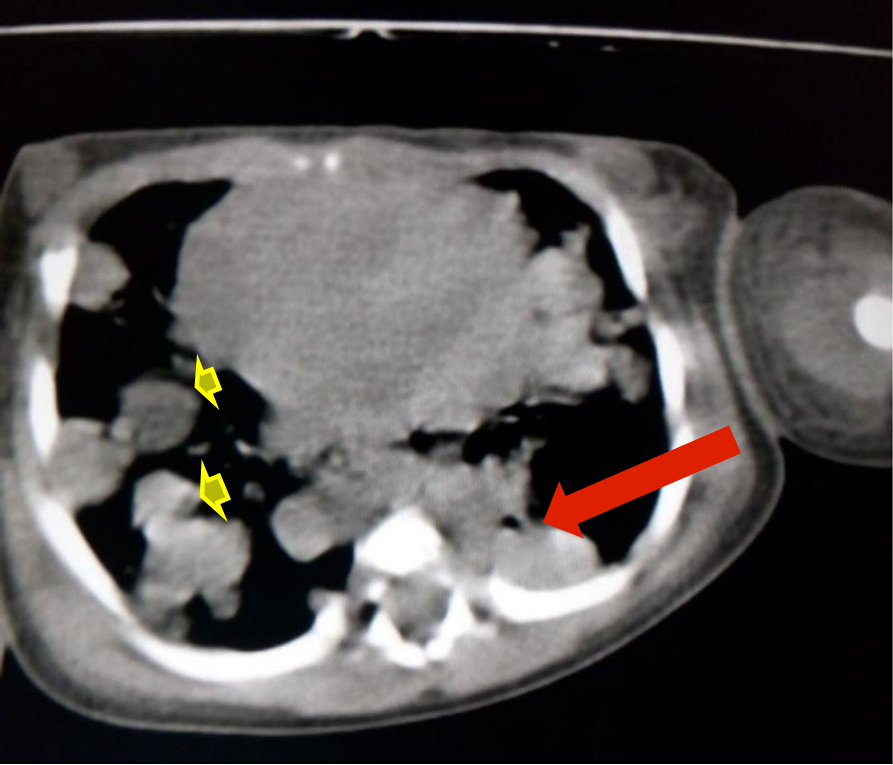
Non contrast computed tomography scan showing soft tissue density lesion in left paravertebral area (*red arrow*) along with multiple lung metastases (*yellow arrowheads*)
